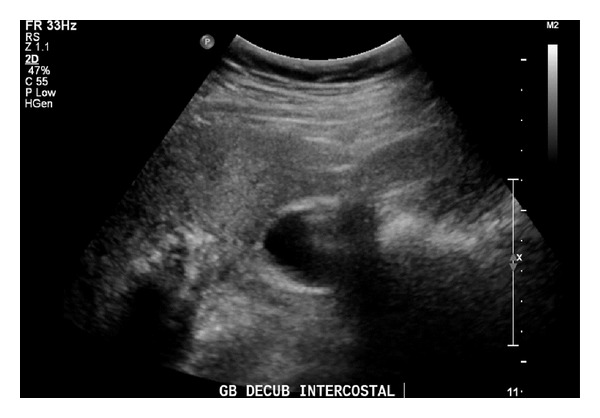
Abnormal gallbladder with thickened wall and mass in the fundus on ultrasound.
